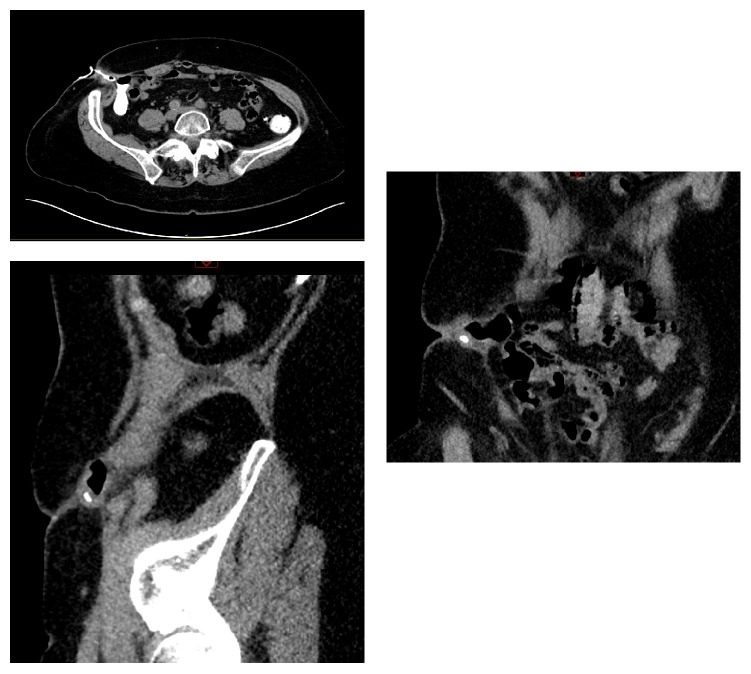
CT images of enterocutaneous fistula.
